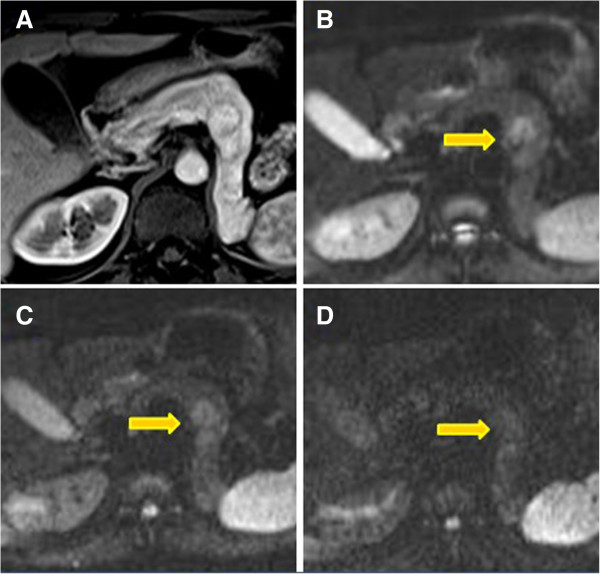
Arterial phase and diffusion-weighted images. Lesions almost isointense to the surrounding pancreatic parenchyma on arterial phase acquisition, circumscribed by a thin hypointense rim (A). They show lower signal intensity on DWI sequences with the increasing of b-values (B, C, D).
